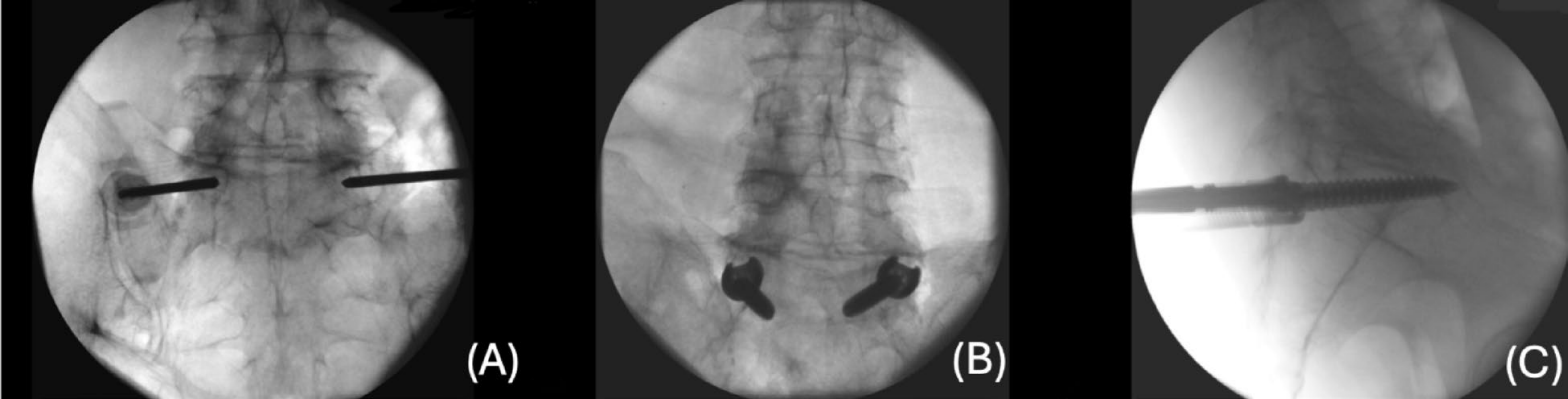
Intraoperative fluoroscopy of percutaneous tricortical S1 pedicle screw fixation. (A) Bilateral Jamshidi needle. Placement demonstrated by an a.p. radiograph, (B) a.p. radiograph confirming correct positions of the pedicle screws. (C) Anterior sacral cortex, exceeded by two threads of the pedicle screw tips. Lateral radiograph.
